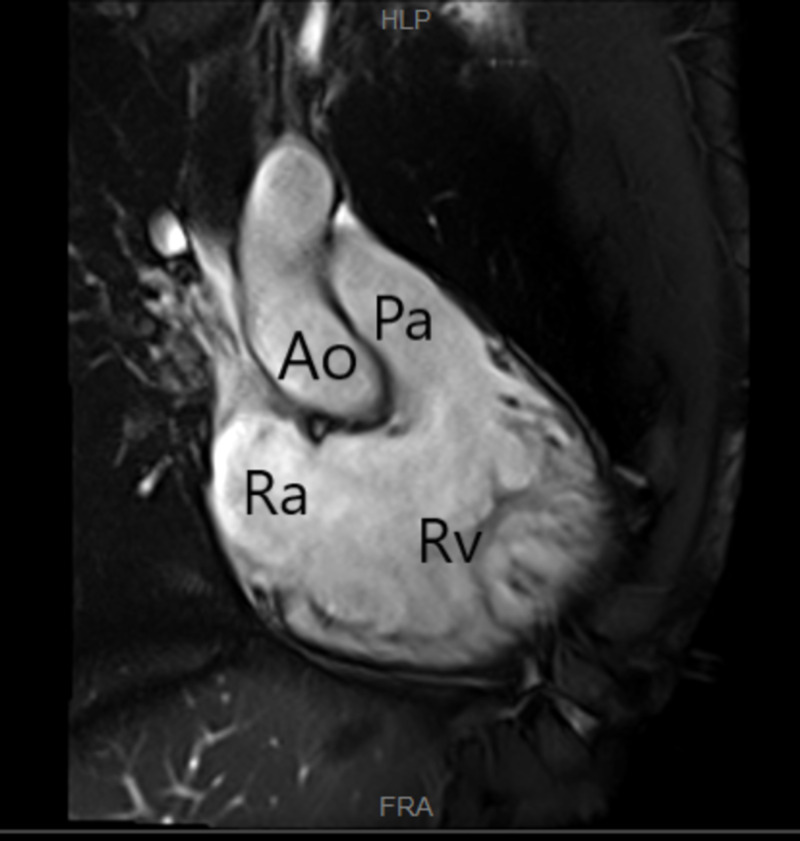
Cardiac MRI white blood imaging, coronal view showing right atrium (Ra), right ventricle (Rv), pulmonary artery (Pa), and aorta (Ao). No evidence of intracavitary thrombus or valvular vegetation.
